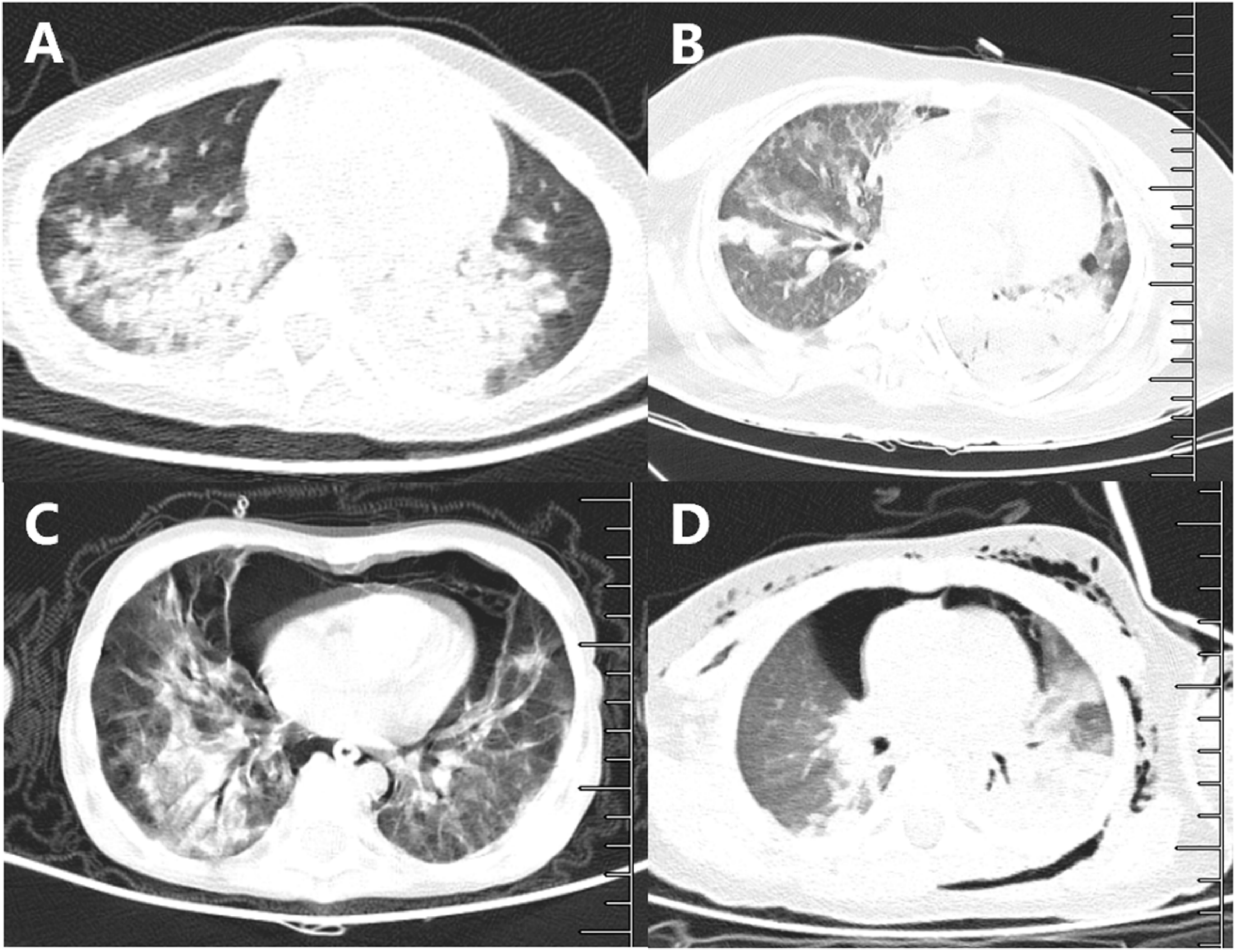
Chest CT findings of acute respiratory distress syndrome children with Omicron variant infection. Chest CT images of 4 critically ill children with ARDS: (A) a 4-year-old girl: ground-glass opacity and large areas of inflammation with partial consolidation; (B) a 16-year-old boy with methylmalonic acidemia; (C) a 6-year-old girl with mediastinal emphysema; (D) a 2-year-old boy with pneumothorax: partial effusion, who was coinfected with EBV.
